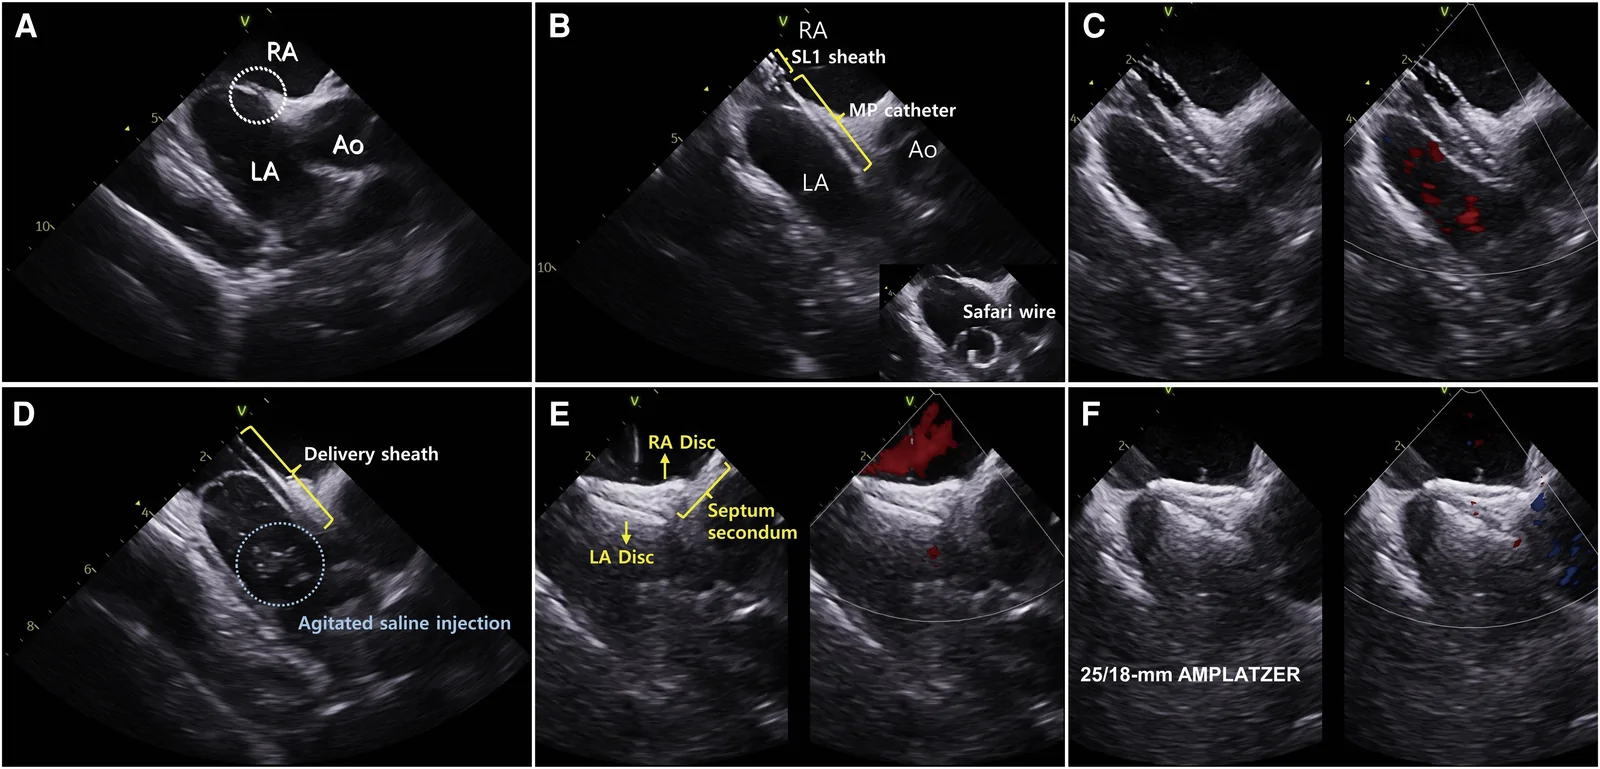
Zero-fluoroscopy, intracardiac echocardiography-only patent foramen ovale closure workflow. (*A*) Initial intracardiac echocardiography examination delineating the patent foramen ovale tunnel. (*B*) Pre-shaped transseptal sheath positioned at the fossa ovalis; inset shows a Safari support wire forming a pre-shaped left atrial rail. (*C*) Stop-flow balloon sizing demonstrating an 11-mm stop-flow diameter. (*D*) Sheath-through agitated saline injection showing dense left atrial microbubble opacification, used as an adjunctive visual check of left atrial position before device release. (*E*) Intracardiac echocardiography confirms left and right atrial discs straddling the septum. (*F*) Final intracardiac echocardiography colour Doppler demonstrates no residual shunt.
